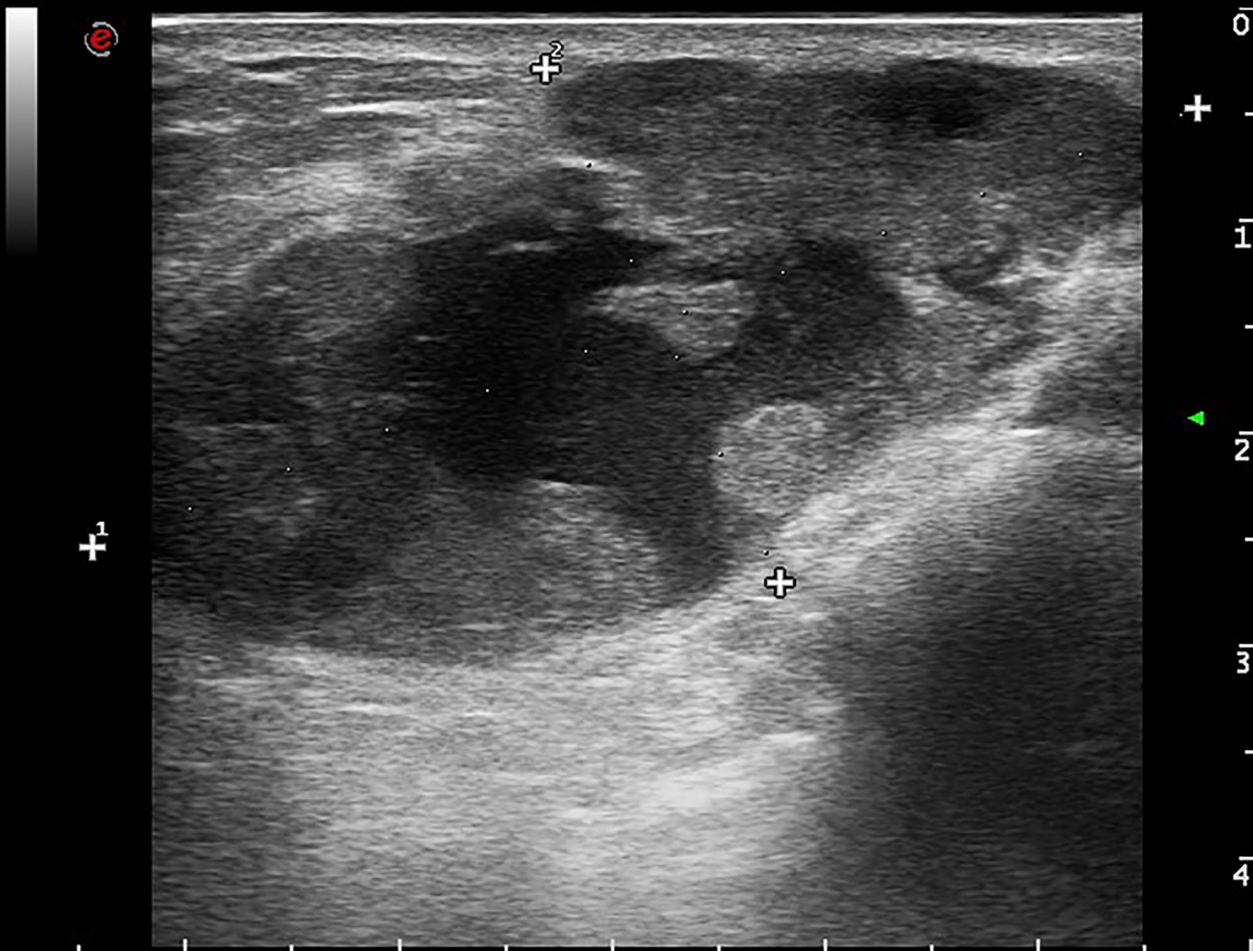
Breast imaging. Ultrasonography shows cystic solid compound echo with a size of 56*44*27mm of the left breast.
